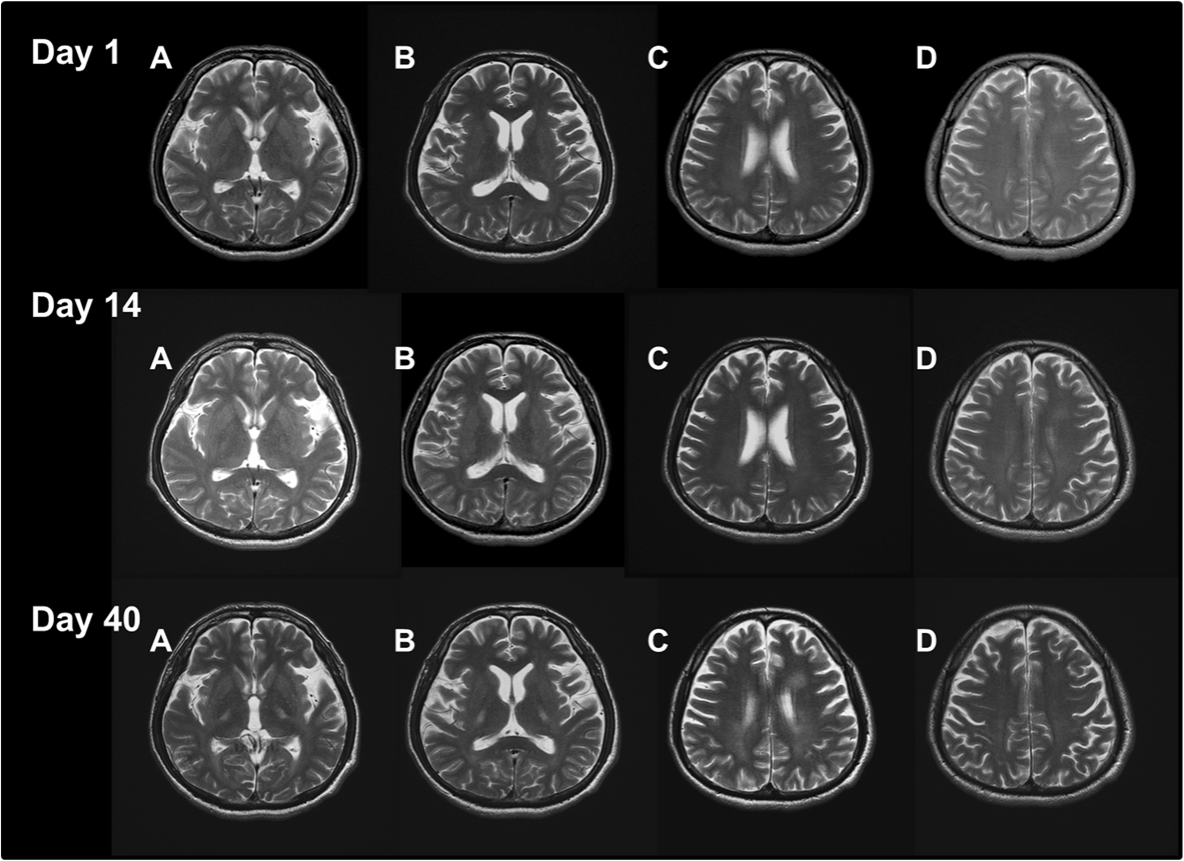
T2-weighted brain MR images on day 1, 14, and 40 after admission. In MR images on day 40, hyperintense foci on T2-weighted images are unequivocally demonstrated in the bilateral internal capsules (Day 40, a and b) and cerebral white matter near the lateral ventricles (Day 40, c)
